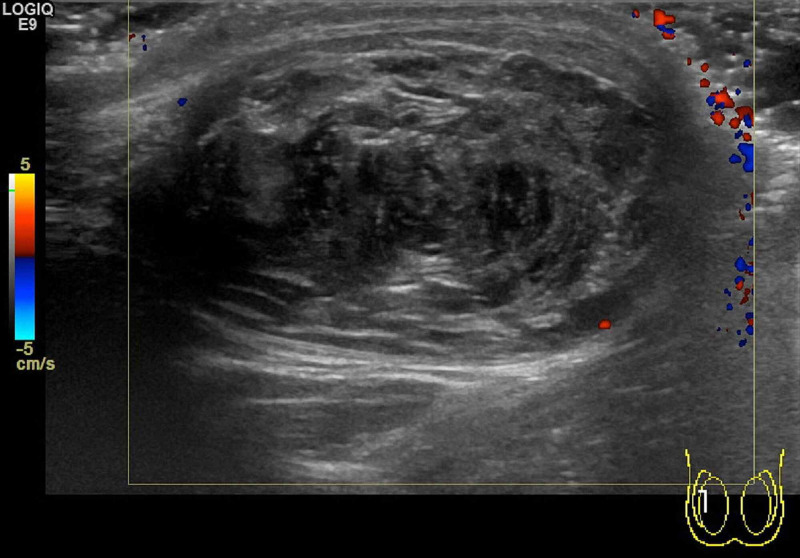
Doppler study of right testicular cyst demonstrating absence of vascular flow.
